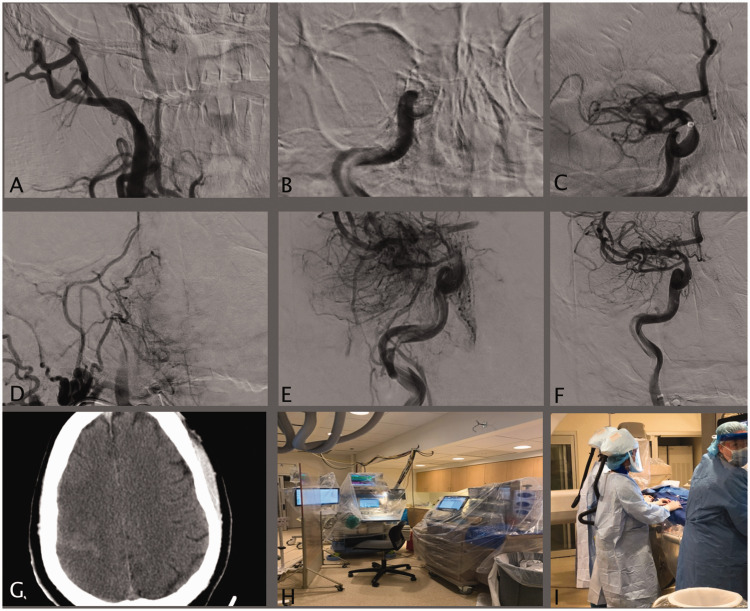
Fifty-one-year-old male with no past medical concerns developed an acute neurological insult. On admission his NIHSS score was 15, head CT did not show any hemorrhage and tPA was given. The patient underwent a MT procedure within 3 h and 52 min. The patient had a right ICA occlusion from the cervical segment into the supraclinoid segment with a tandem M1 occlusion. The procedure was complicated by ICA re-occlusion and distal embolization occluding the A1. The insult progressed into complete infarct, and the patient passed away three days later. (a) Antero-posterior (AP) digital subtraction angiography (DCA) of a right ICA injection showing complete occlusion at cervical ICA segment; (b) AP DCA view showing an occlusion of the supraclinoid segment of the ICA; (c) AP DCA view showing an M1 occlusion; (d) AP DCA view showing re-occlusion of the cervical ICA segment; (e) AP DCA occlusion of the A1 segment either due to a distal embolization or a dissection; (f) final view showing revascularization of the ICA, M1, and A1 with a TICI 2B result by deploying intracranial and extracranial rescue stents; (g) non-contrast axial view of the brain, day 1 post mechanical thrombectomy showing the progression of the insult into a complete infarct; (h) all the devices in the room are draped; and (i) showing the powered air-purifying respirator used by operators.
